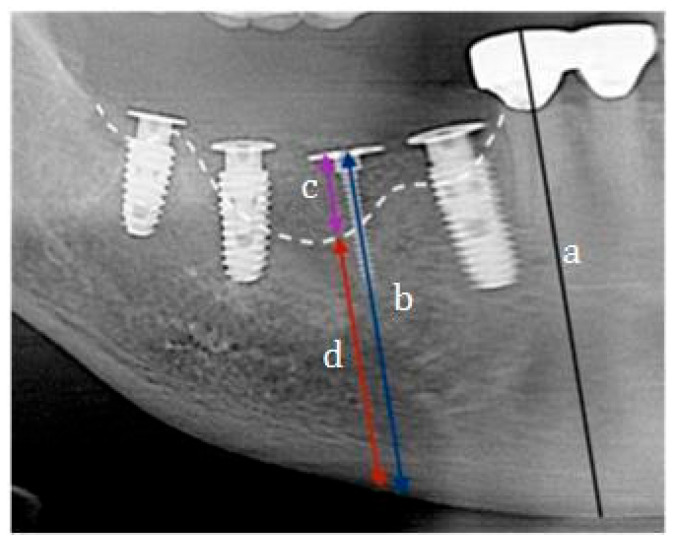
Postoperative radiographic measurement of subject (a) Reference line: A baseline drawn parallel to the long axis of the adjacent teeth. (b) Measurement 1: Total bone height measured from the lowest point of the mandible to the top of the wide-head tent-pole screw. (c) Measurement 2: Augmented bone height measured from the top of the tent-pole screw to the graft junction, where the native mandibular bone meets the grafted bone. (d) Original bone line: Pre-augmentation bone height measured from the mandibular base to the graft junction.
